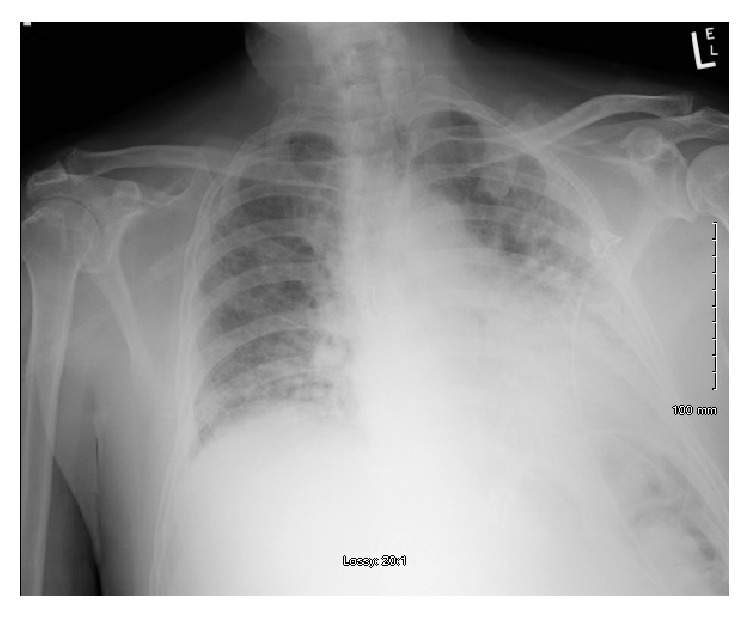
Chest X-ray showing worsening reticular and hazy markings throughout bilateral lung fields compared to the previous chest X-ray.
